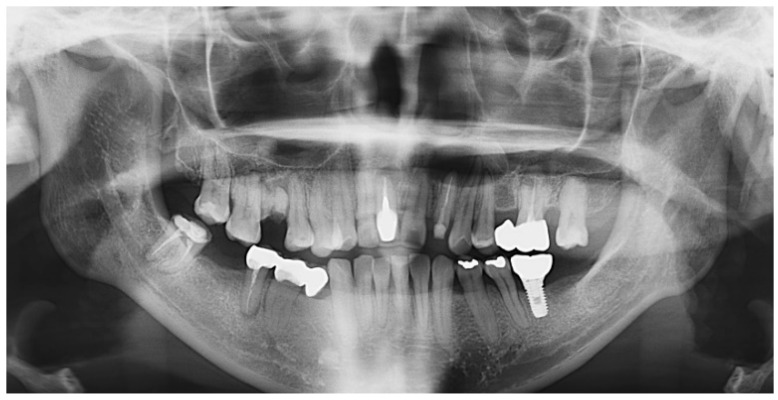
Initial panoramic X-ray. Pre-operative radiographs revealed a cystic lesion on the left anterior maxilla, and there were two teeth to be extracted: right maxillary first molar and left maxillary third molar, respectively.
